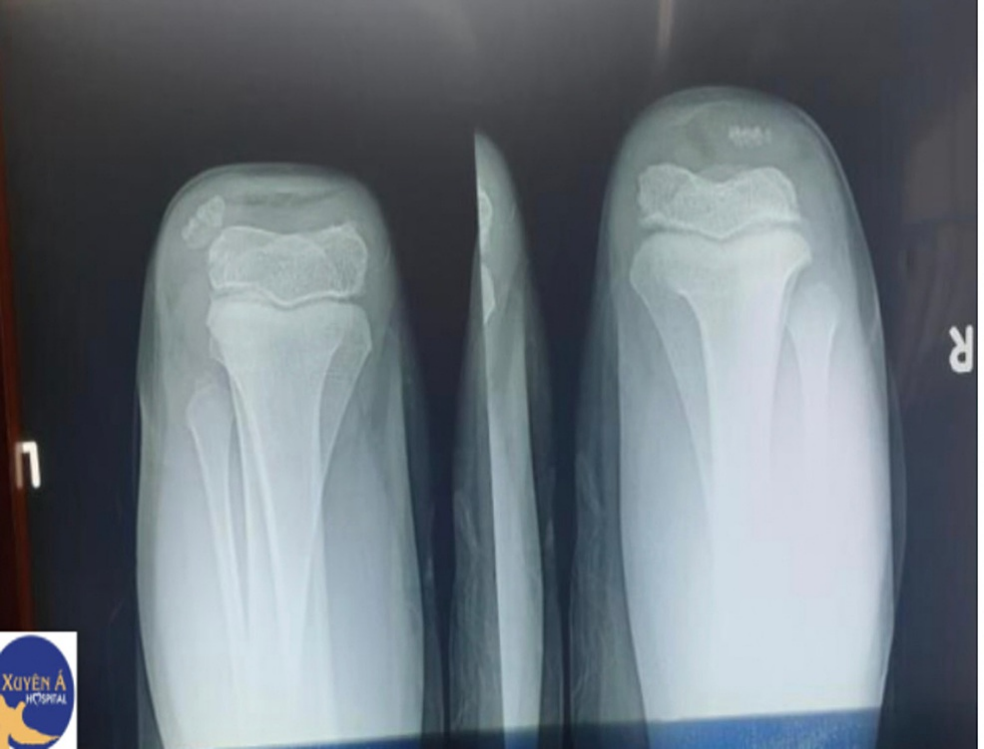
X-ray reveals dislocated patella on the left knee
